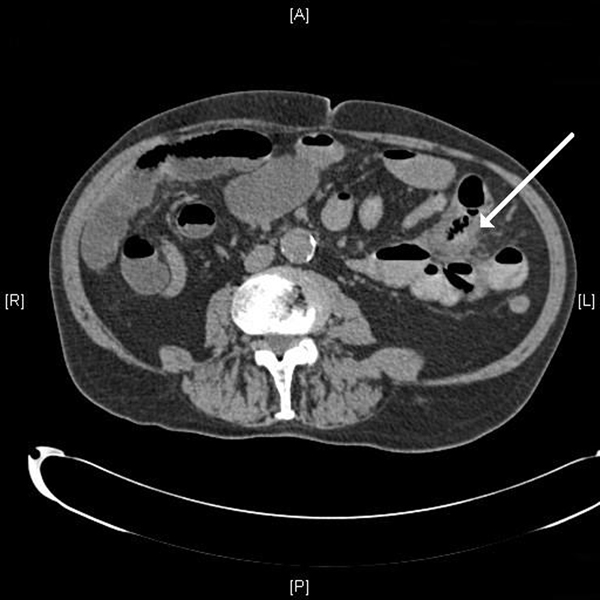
Computed tomography scan demonstrating marked soft tissue stranding containing multiple small locules of gas and which is surrounded by several small bowel loops (arrow). This area appears to connect to a loop of small bowel but does not definitely lie in continuity with the rest of the bowel, which is very suspicious for a localised perforation of the small bowel.
